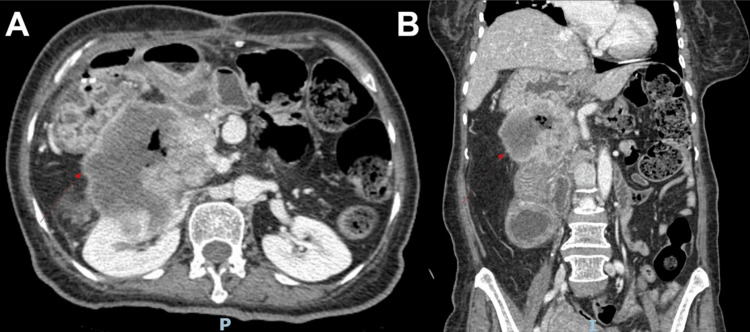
CT scan of the abdomen and pelvis revealed disease progression: (A) Axial view, (B) Coronal view.
